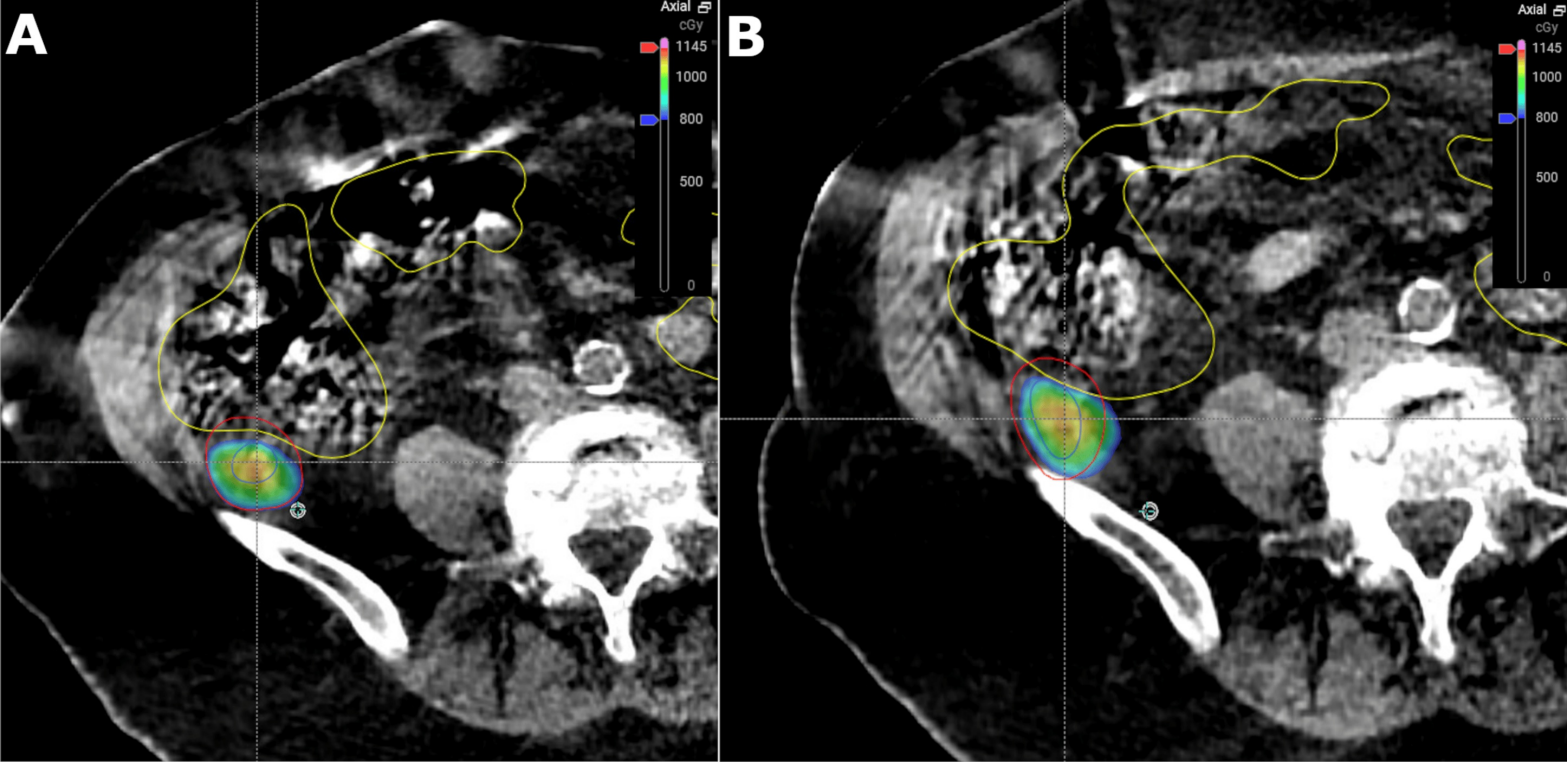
CBCT-based online adaptive plan-of-the-day for the (A) first fraction and (B) fourth fraction, with a color wash demonstrating differences in coverage of the retrocolic GTV (blue) and PTV (red) by the prescription dose, due to variations in the proximity of the colon (yellow) CBCT: cone-beam computed tomography; GTV: gross tumor volume; PTV: planning target volume
